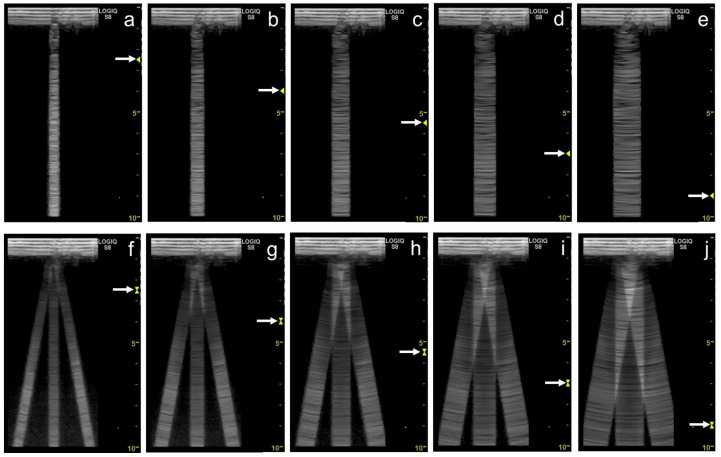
Ultrasound images obtained with the. linear transducer. The vertical artifact becomes wider as the focal point (arrow) is moved to deeper levels (a–e). When spatial compound imaging is enabled, the single vertical artifact changes to three lines radiating from the same point (a,f). The three lines become wider as the focal point (arrow) is moved to deeper levels (f–j).
